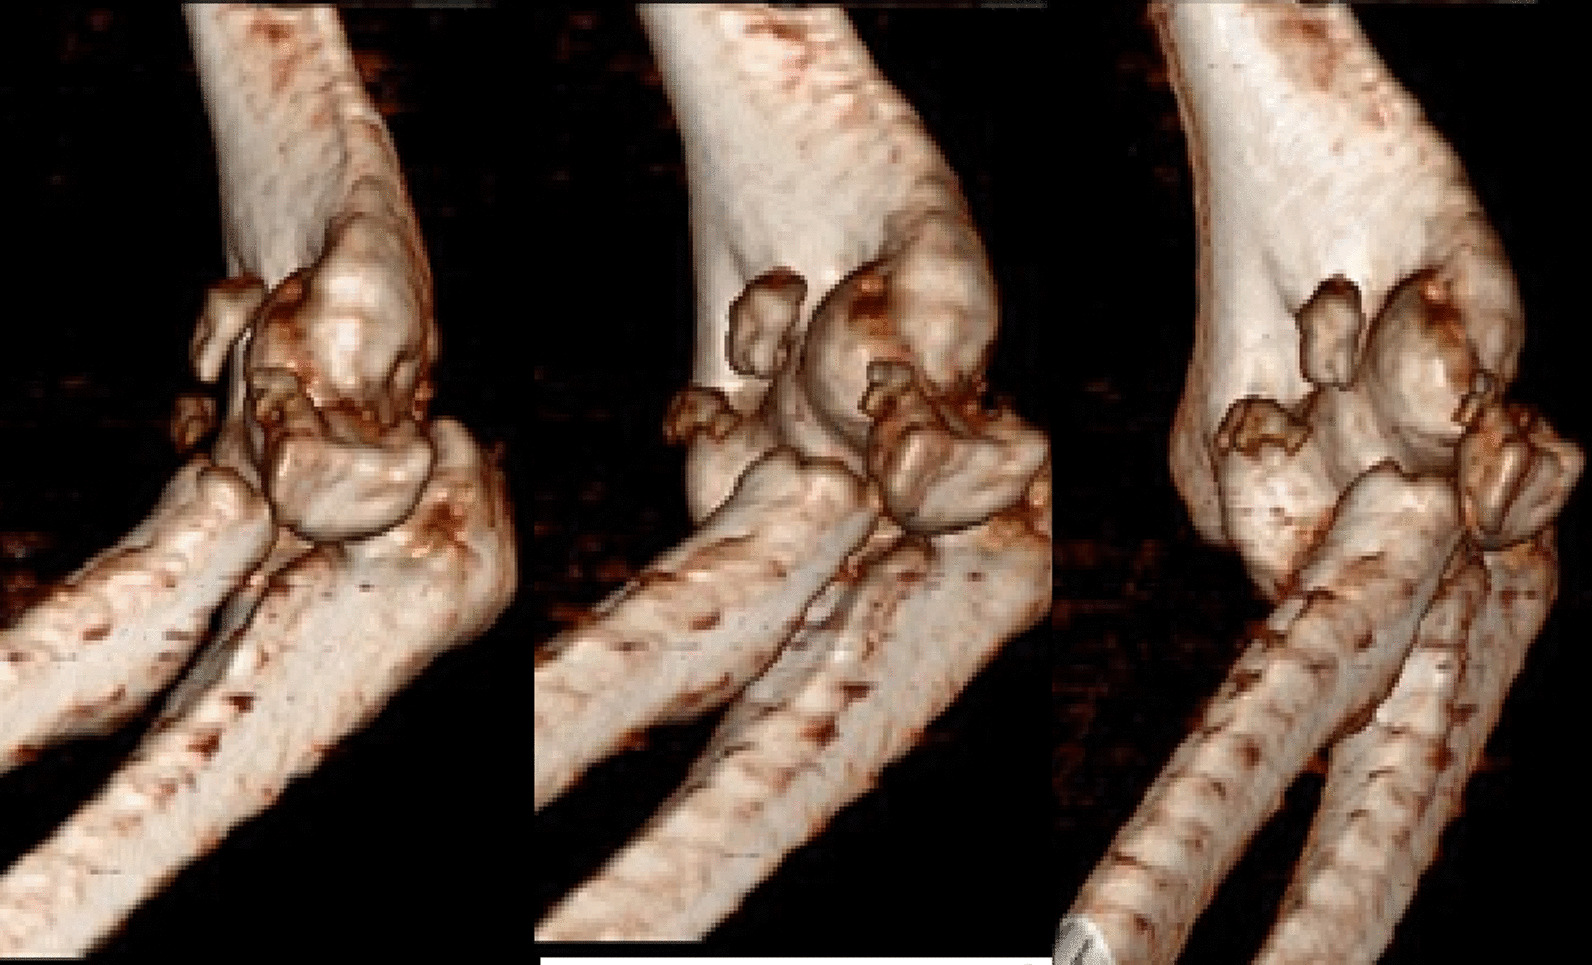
Left elbow computed tomography (CT) imaging; radial head comminution (Mason type 4) and coronoid fracture (Regan–Morris type 1) are described in great depth
